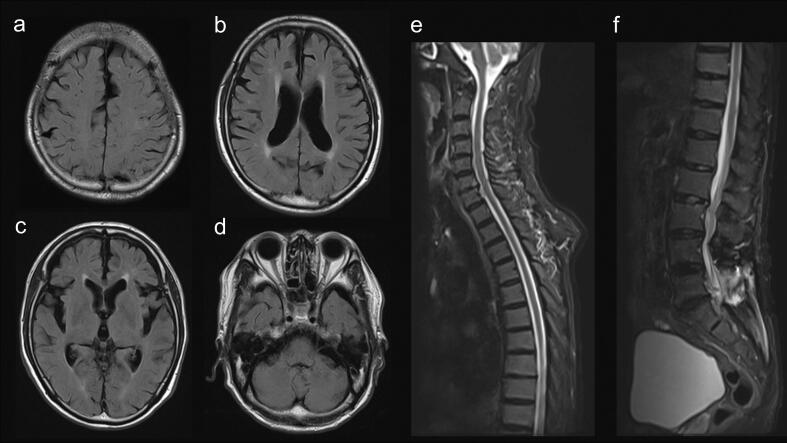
Brain and spine MRI. FLAIR MRI of the brain and T2WI MRI of the spine. Brain and spinal cord lesions are not shown (a-f). Mild stenosis in L2-4 and post-surgical signal changes in L5-S1 are shown (e, f).
